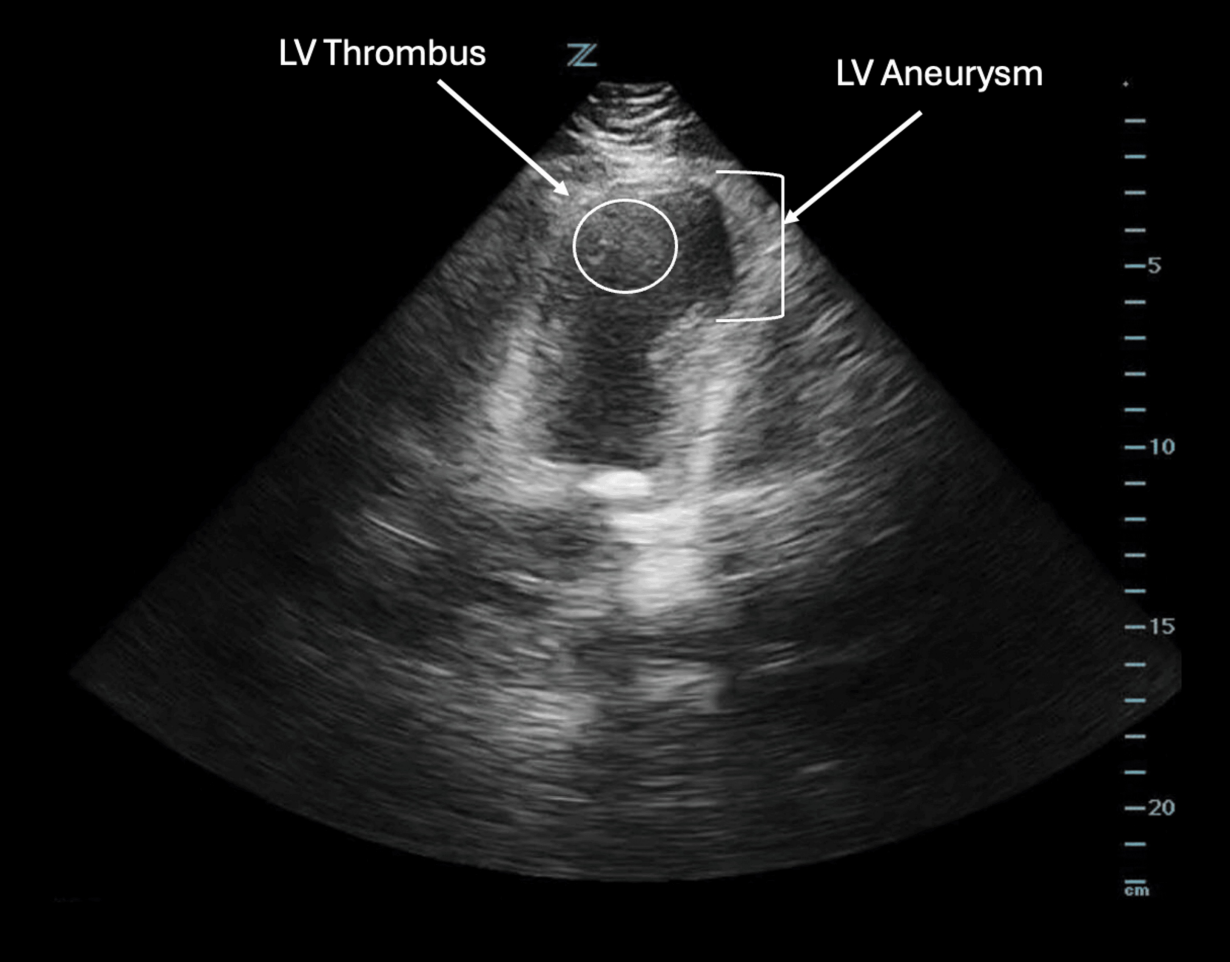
An apical four-chamber view of the left ventricle in Case 2, obtained using a phased array probe, demonstrating left ventricular aneurysm with a left ventricular thrombus (outlined in white circle).
